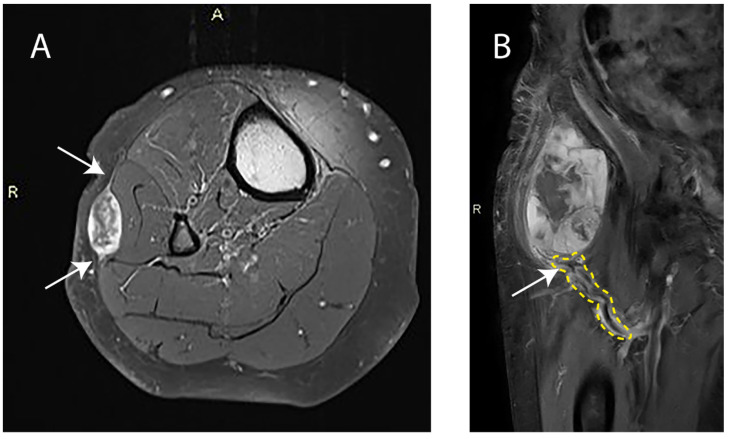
(A) Axial-contrast-enhanced T1-weighted image with fat saturation of a right lower leg with a superficial myxofibrosarcoma. Arrows indicate the tail sign. A: Anterior orientation; R: Right side orientation. (B) Coronal-contrast-enhanced T1-weighted image of a right upper leg with a deep myxofibrosarcoma. The area outlined in yellow highlights the pathologic tumor-feeding vasculature and the arrow indicates the vascular pedicle. R: Right side orientation.
